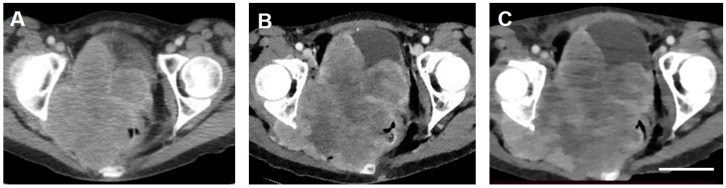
Transverse computed tomographic images of the Stage IIB carcinoma of the cervix obtained from the patient before (A) and 3 (B) and 21 days (C) after treatment with TOL. The images were chosen to depict cuts through the tumor at levels as closely similar as possible. Adjustments of magnifications were made to match the dimensions of bony landmarks of the femoral head and acetabulum. Adjustments of contrast and brightness for optimum clarity of the images were applied equally to all the three images. Note that the size of the tumor increases with each successive image. By contrast-enhanced CT, areas of hypodensity observed within the tumor mass appear larger and much more prominent after treatment with TOL, consistent with the reduction in measured tissue densities from 70 HU pre-treatment (A) to 56 HU (B) by day 3 and 47 HU (C) by day 21 post-treatment. Additional region-of-interest (ROI) reference measurements were made for each scan over the left gluteal musculature revealing values of 127 HU (A), 120 HU (B) and 125 HU (C), respectively. Calibration bar in C = 5 cm.
